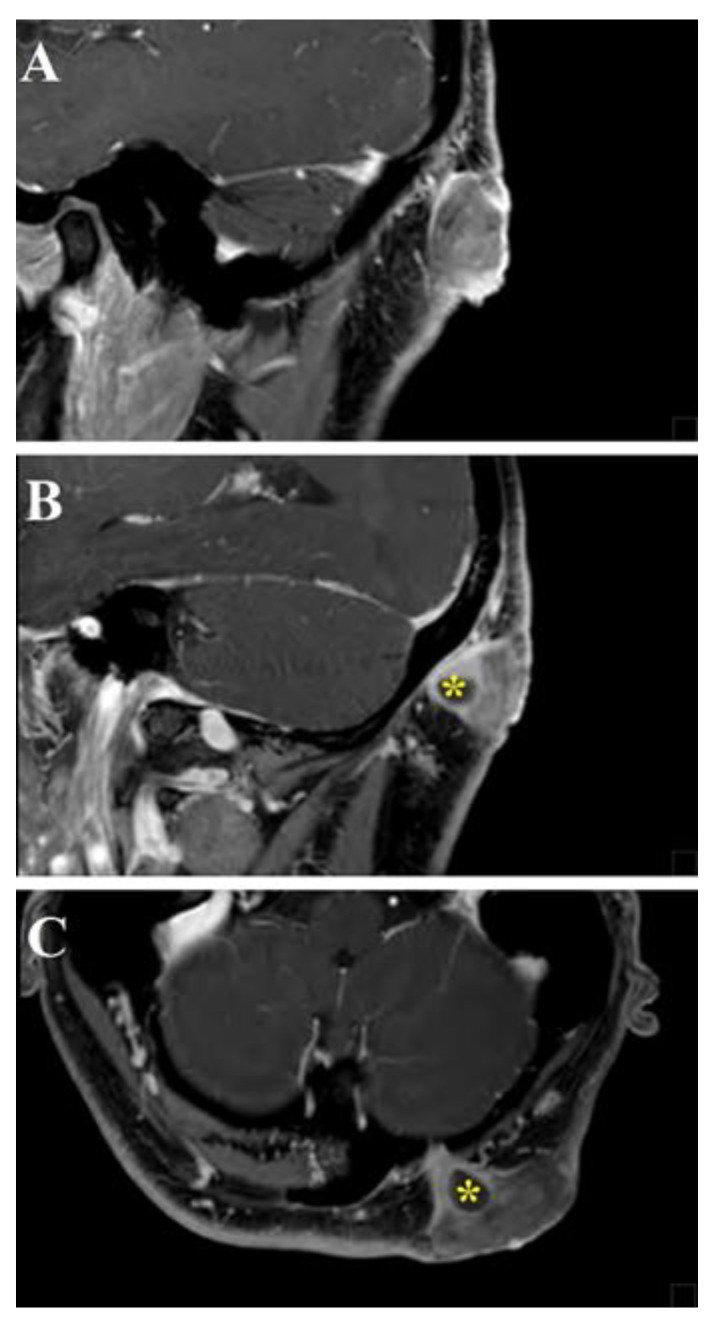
Head and neck MRI sagittal (A,B) and axial (C) images showed a cutaneous mass characterized by homogeneous post-gadolinium enhancement, isointense in T1-weighted sequences, with a characteristic sinus-like propagation that connected the tumor with the cerebellar dura mater through a small passage in the left occipital bone (yellow asterisks).
